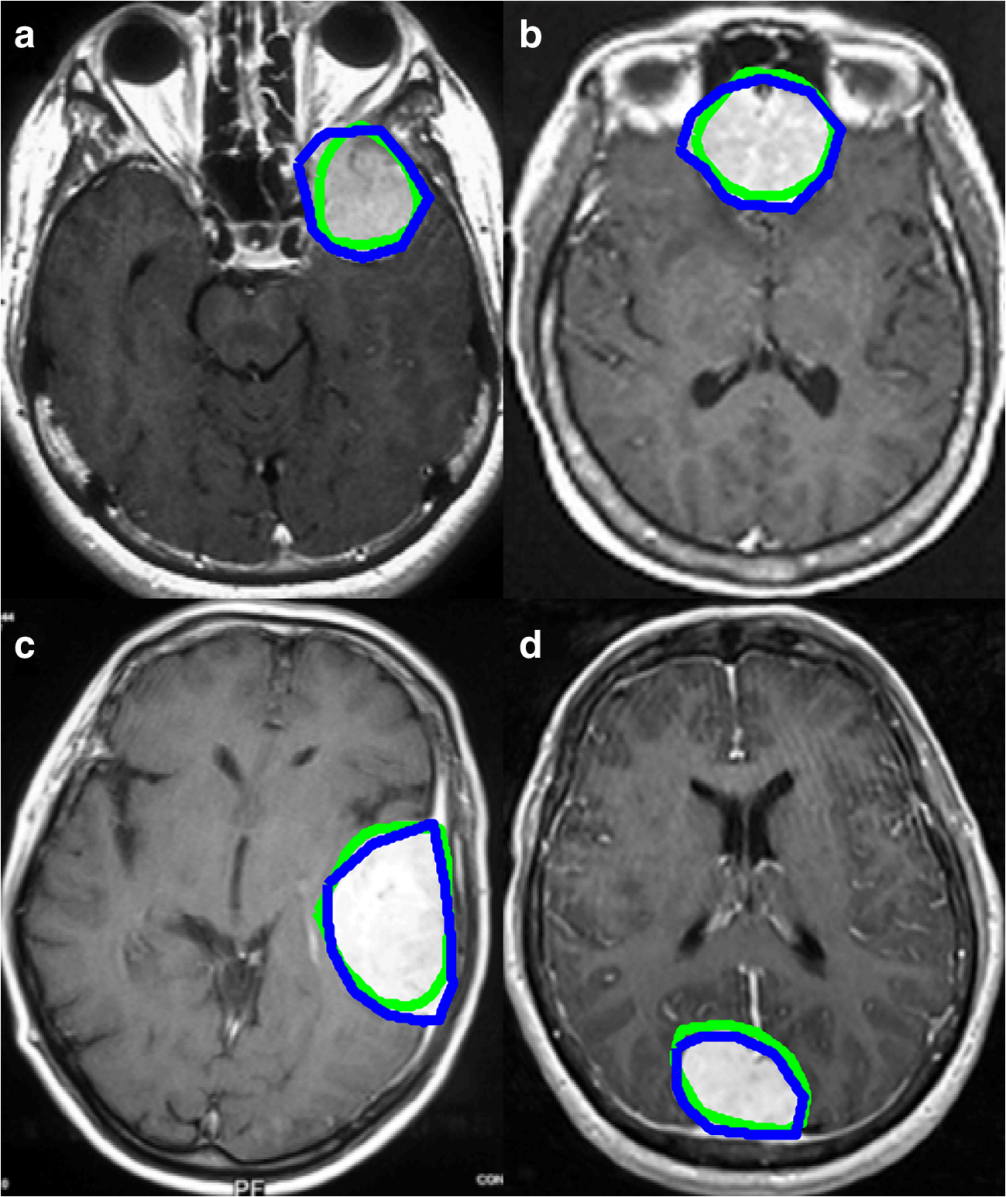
Four examples of meningioma mask border comparisons. Eye-tracking (green) and hand annotation (blue) mask contours are overlaid with the corresponding postcontrast T1-weighted images containing meningiomas
